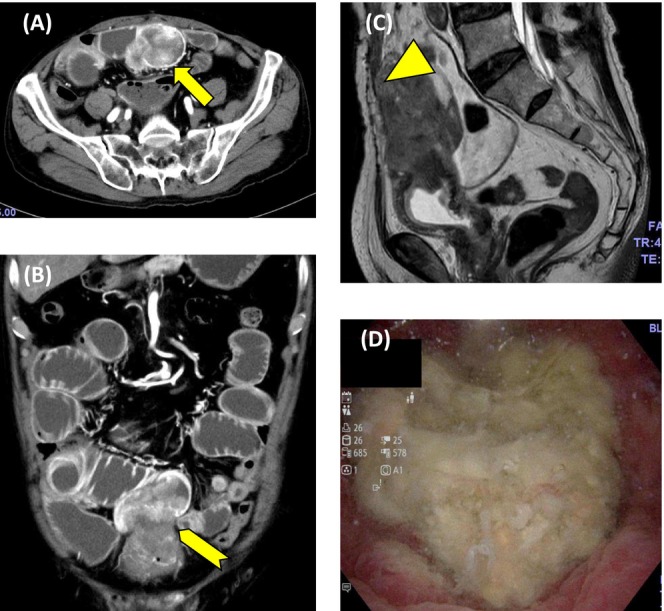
Contrast‐enhanced CT revealed a peripherally enhancing mass (A, arrow), which involved the dome of the bladder and the ileum (B, arrowhead). Magnetic resonance imaging suggested that the mass invaded the rectus abdominis (C, triangle). Cystoscopy showed a necrotic mass at the dome of the bladder (D).
